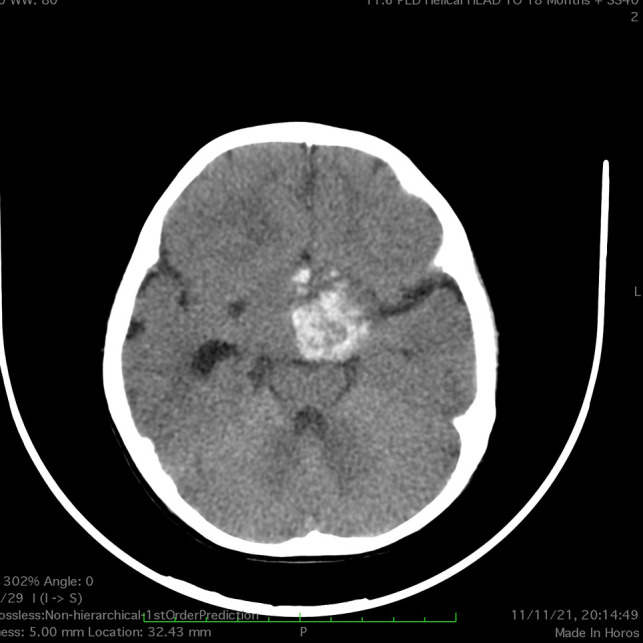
CT scan of the brain (case 2) reveals a large hyperdense suprasellar lesion and mild ventricular dilatation.
